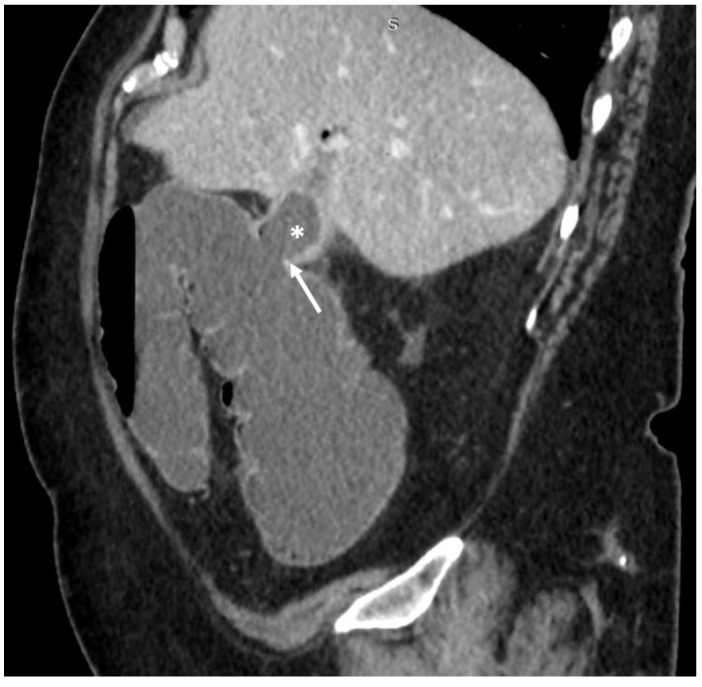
69-year-old female. Abdominal CT scan, sagittal plane, portovenous phase. Collapsed gallbladder (asterisk), there is a defect in the wall connecting the lumen of the gall bladder with the lumen of hepatic flexure of the colon–cholecystocolic fistula (arrow).
